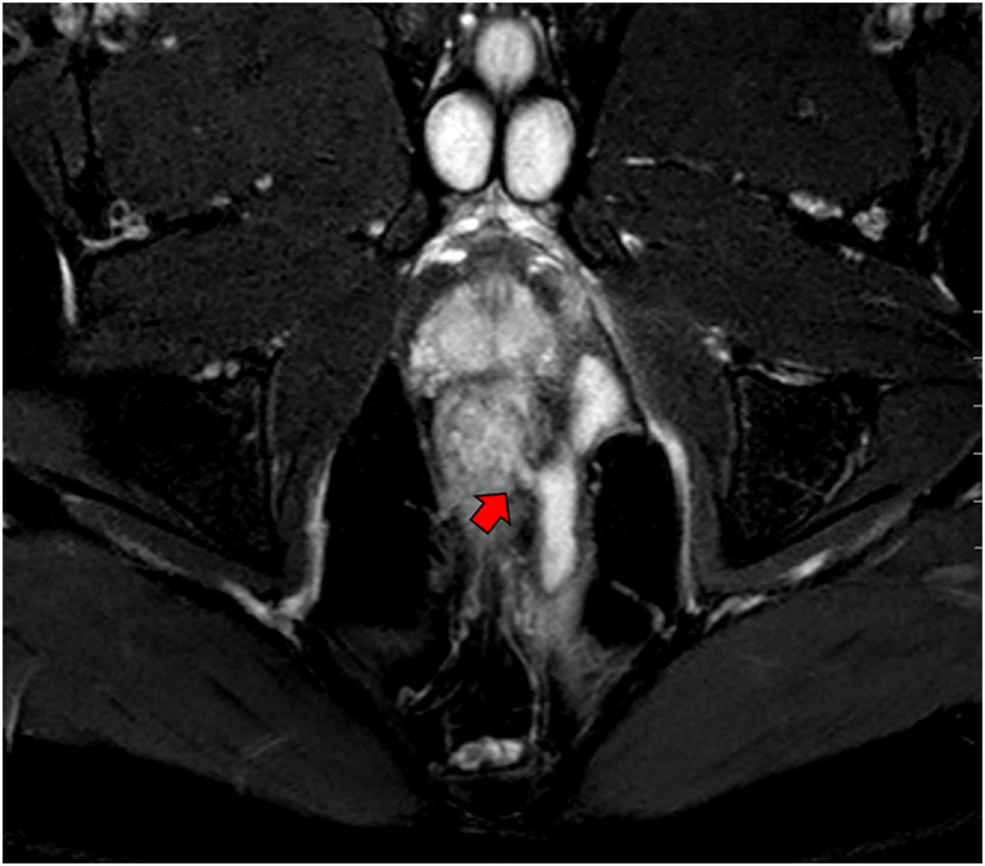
Complex perianal fistula with clear internal orfice (arrow). Coronal T2-weighted post-contrast MRI
